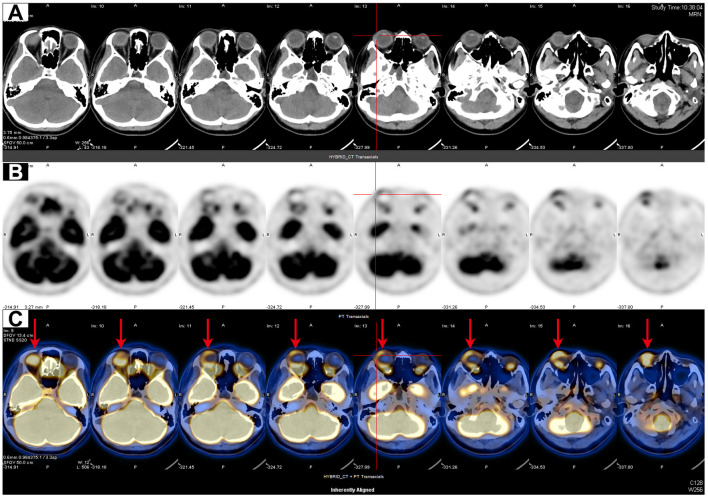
PET/CT scan of the orbit. (A–C) Axial orbital CT (A), PET (B), and fusion PET/CT (C) images showed thickening of the ocular ring wall with increased FDG uptake in the right eye (red arrows).
